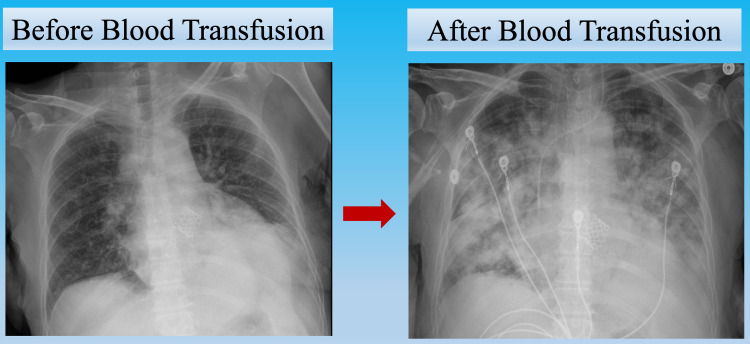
A comparison of chest X-ray: Pre- and Post- transfusion
